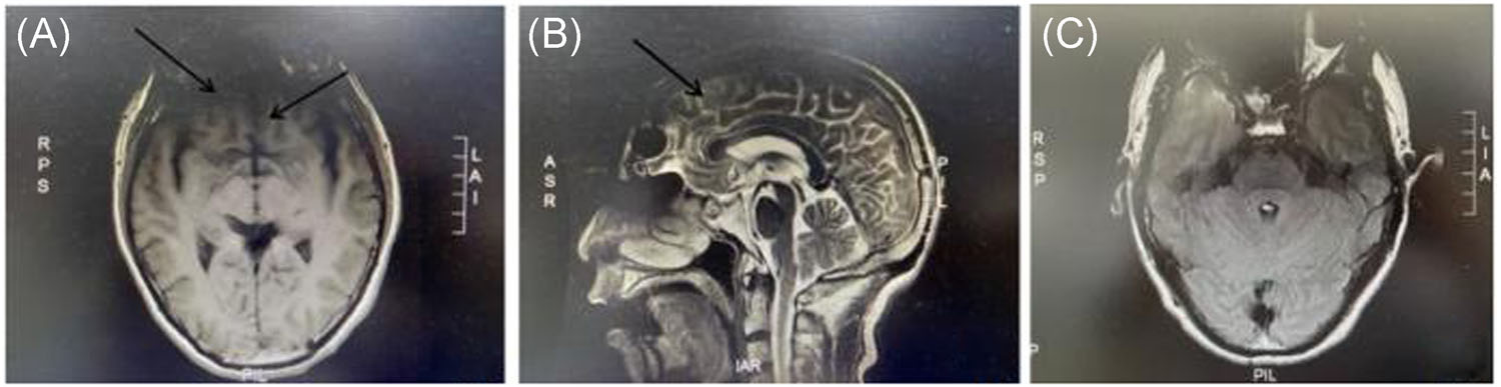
Cranial MRI examination. The sulcus near the knee of the corpus callosum widens (A, arrow), unclear display of some structures in frontal lobe (B, arrow), FLAIR image examination (C) showed no abnormal signal in hippocampus and amygdala. MRI, magnetic resonance imaging [Color figure can be viewed at wileyonlinelibrary.com]
